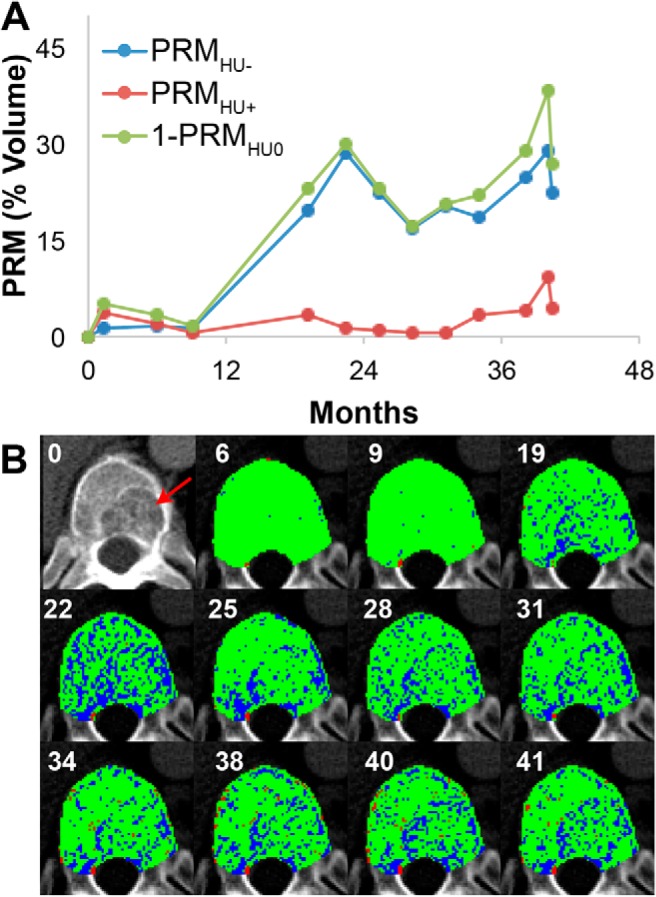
An osteolytic lesion (T11) was visible on the initial CT scan, and subsequent longitudinal PRM analysis shows a substantial reduction in bone density at around 20 months that was visible as a diffuse pattern of PRM_HU−_ (blue).
